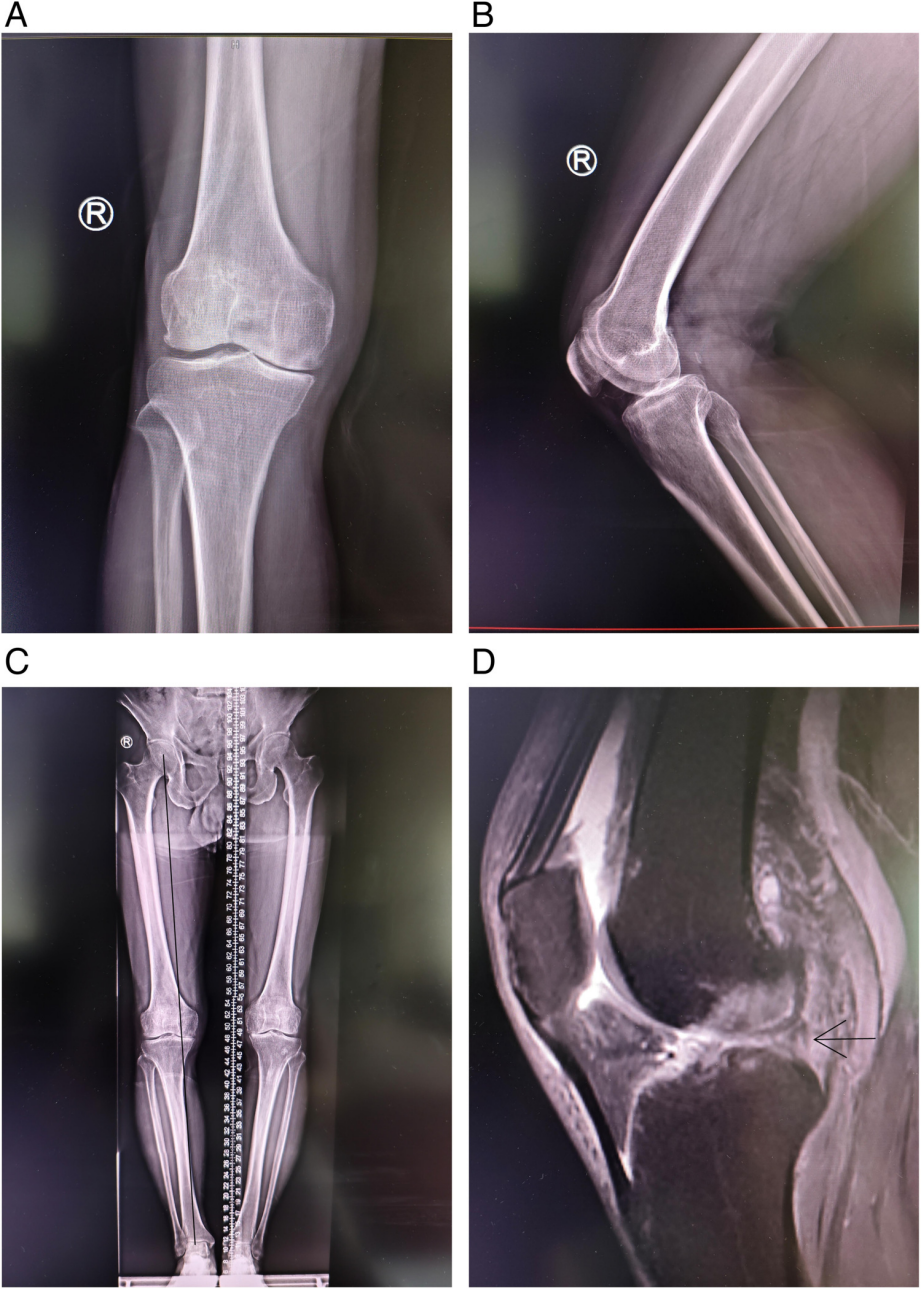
Preoperative X-ray and MRI scans of the right knee joint. (A,B) Anteroposterior and lateral X-ray; (C) the right lower limb was markedly varus; (D) MRI revealed a tear in the posterior root of the lateral meniscus (marked by red arrow). MRI, magnetic resonance imaging.
